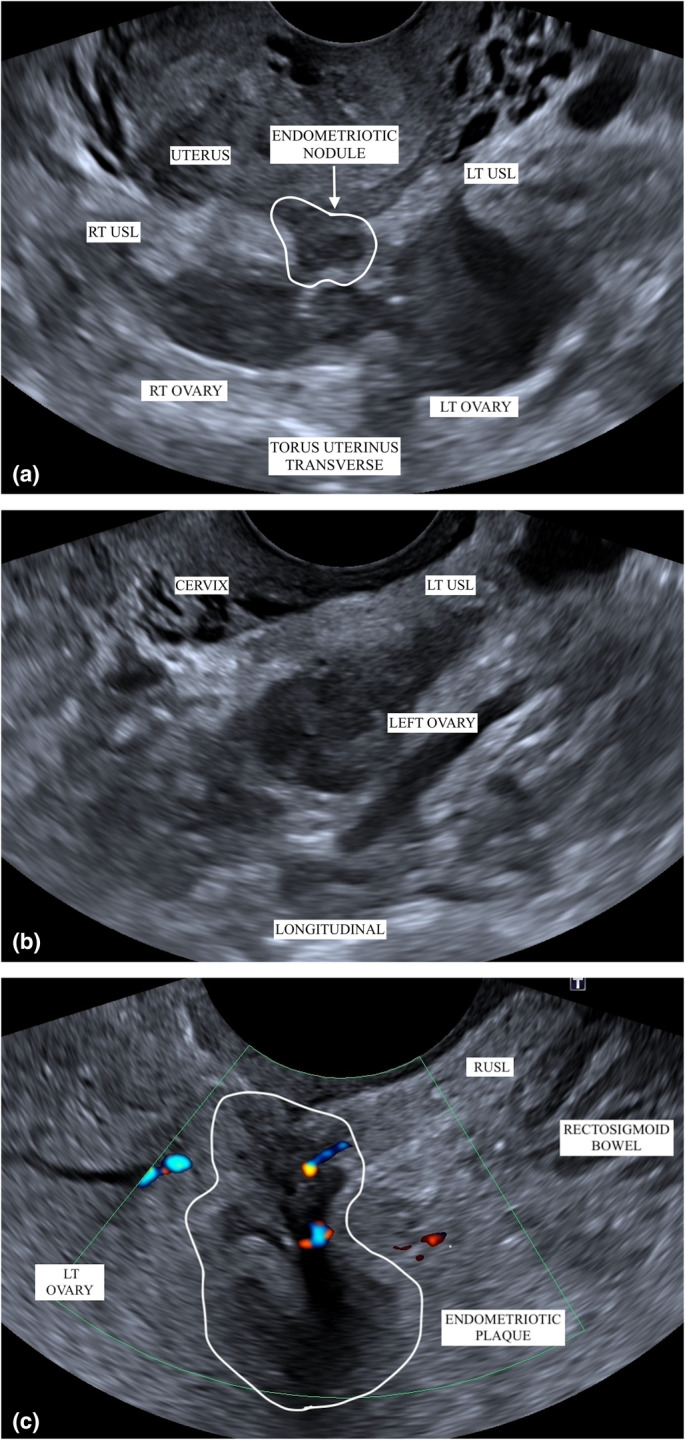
Laparoscopy diagnosed endometriosis with TVS appearances of DE. (a) Transverse plane of bilateral ovarian endometrioma with medialisation of both ovaries adherent to the USLs and the uterine fundus. There is the appearance of an endometriotic nodule at the TU involving both USLs. (b) The LUSLs demonstrated longitudinally is seen thickened, heterogeneous and irregular with the left ovaried adhered. (c) Frozen pelvis, with extensive deep infiltrative endometriosis where the patient report focal sharp pain during sonopalpation. There is endometriosis plaque around the level of the rectosigmoid bowel with appears tethered to the inferior margin of the ovaries, cervix and extends to the right USL with tethering. DE, deep endometriosis; TU, torus uterinus; TVS, transvaginal ultrasound; USL, uterosacral ligaments.
